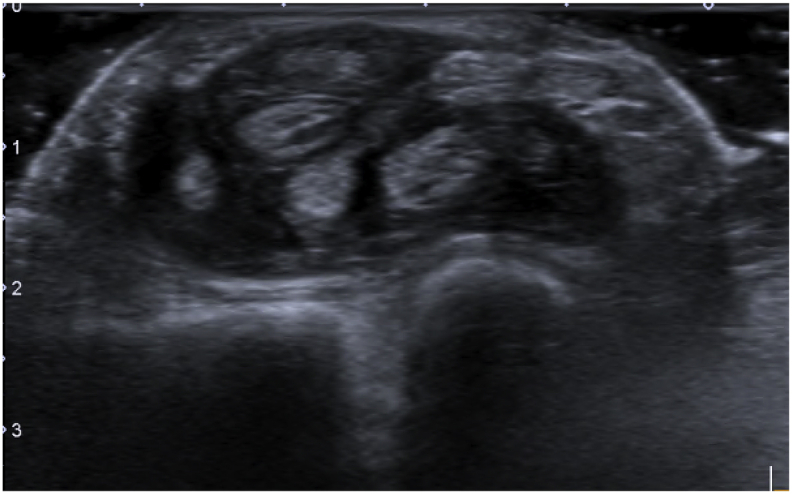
Transverse ultrasound view at wrist level showing thicke ning of the synovium and flexor tendon.
